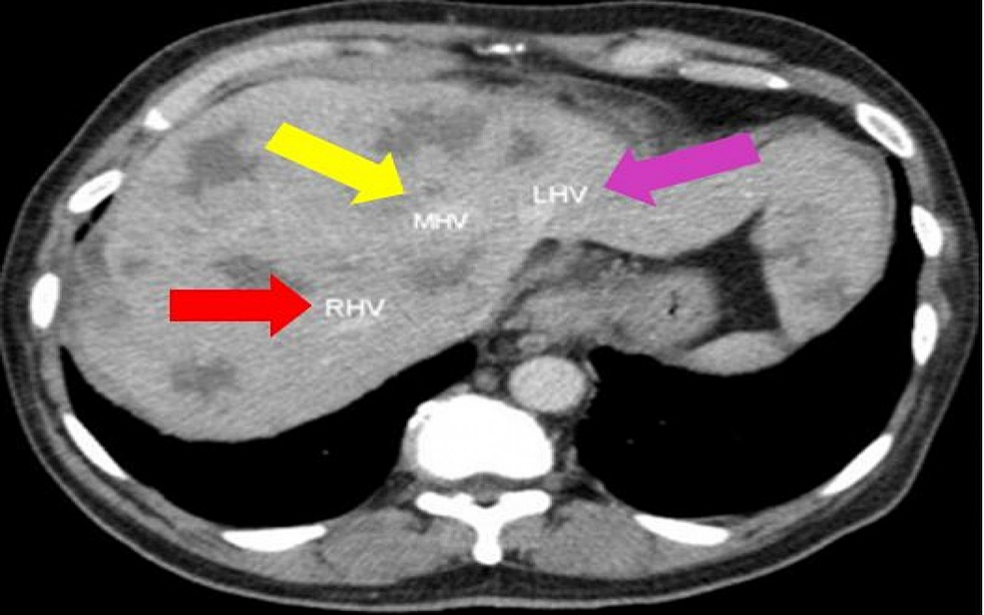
CT cross-sectional view of the abdomen shows widespread metastasis of stomach cancer to the liver and portal area. The arterial phase demonstrates an early enhancement CT: computed tomography; MHV: middle hepatic vein (yellow arrow); RHV: right hepatic vein (red arrow); LHV: left hepatic vein (pink arrow)
